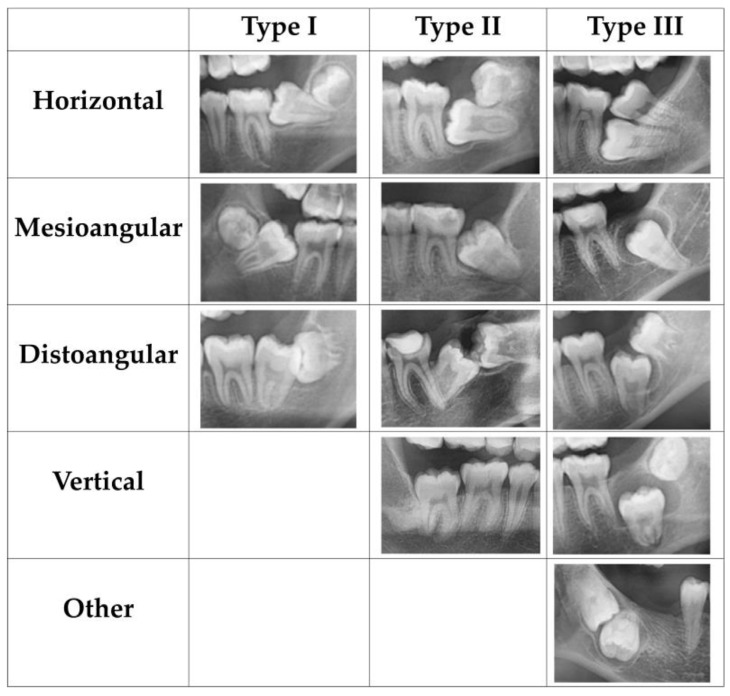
Panoramic radiographs with different angulations of MM2 between the three types.
